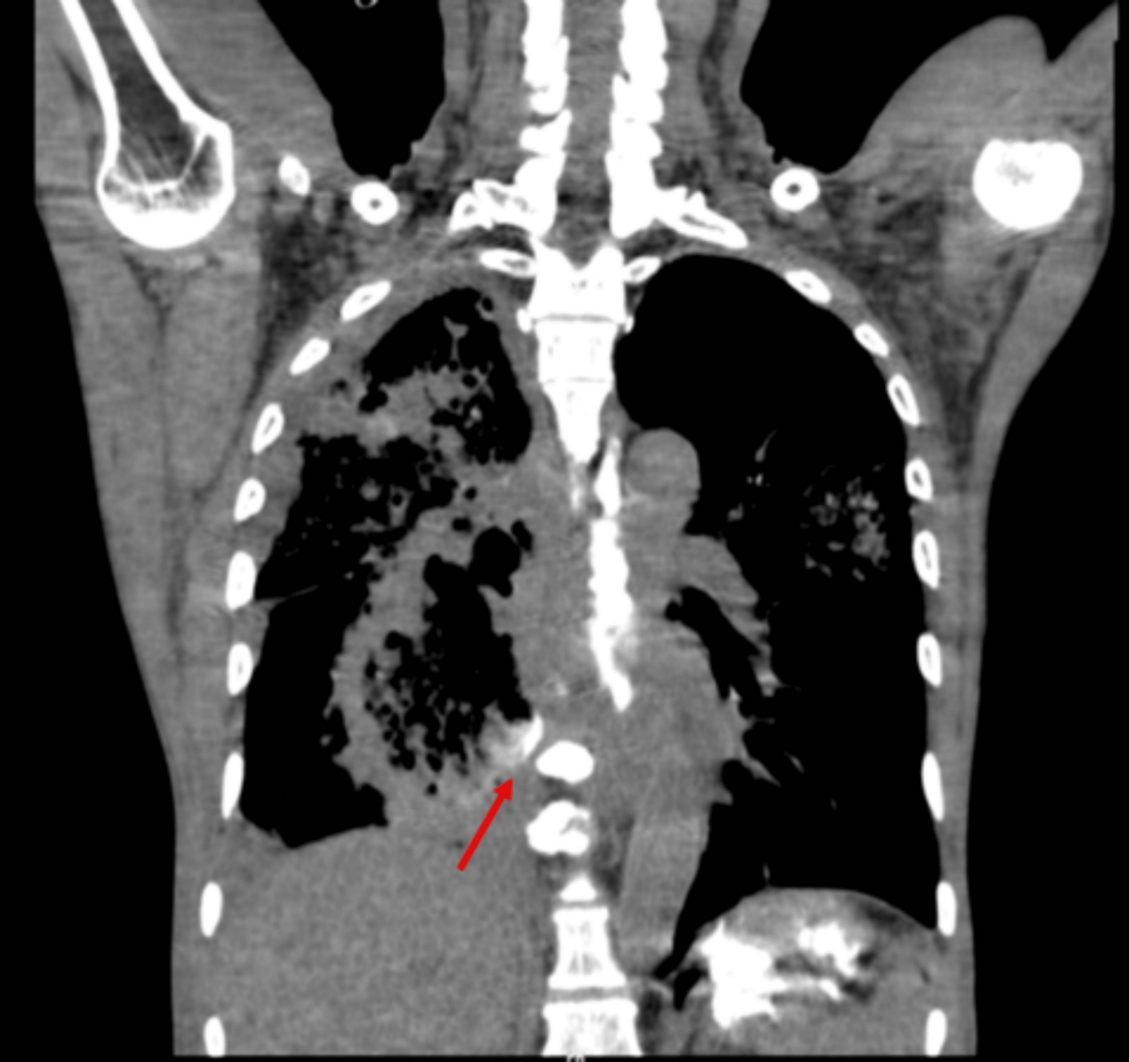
CT oral contrast phase - coronal section The red arrow shows contrast leak into the right lung parenchyma.
